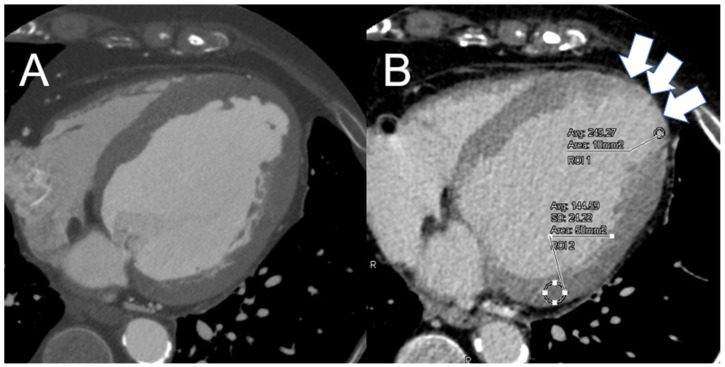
Quantitative image quality analysis. (A) Axial early contrast phase cardiac computed tomography image; (B) Axial late contrast phase cardiac computed tomography image in the same case as (A). Quantitative image quality analysis was conducted by placing regions of interest (ROIs) measuring approximately 10 mm^2^ in the LE regions and 50 mm^2^ in the remote myocardium without late enhancement (LE). The ROIs were manually selected in the LE areas (white arrows in (B), ROI 1) and the remote normal myocardium (ROI 2 in (B)). Computed tomography (CT) attenuation values were measured in patients showing LE in the LVM in CT. The contrast-to-noise ratio (CNR) was calculated as the difference in attenuation between the LE and remote normal myocardium, divided by the standard deviation (SD) of the attenuation in the remote normal myocardium.
